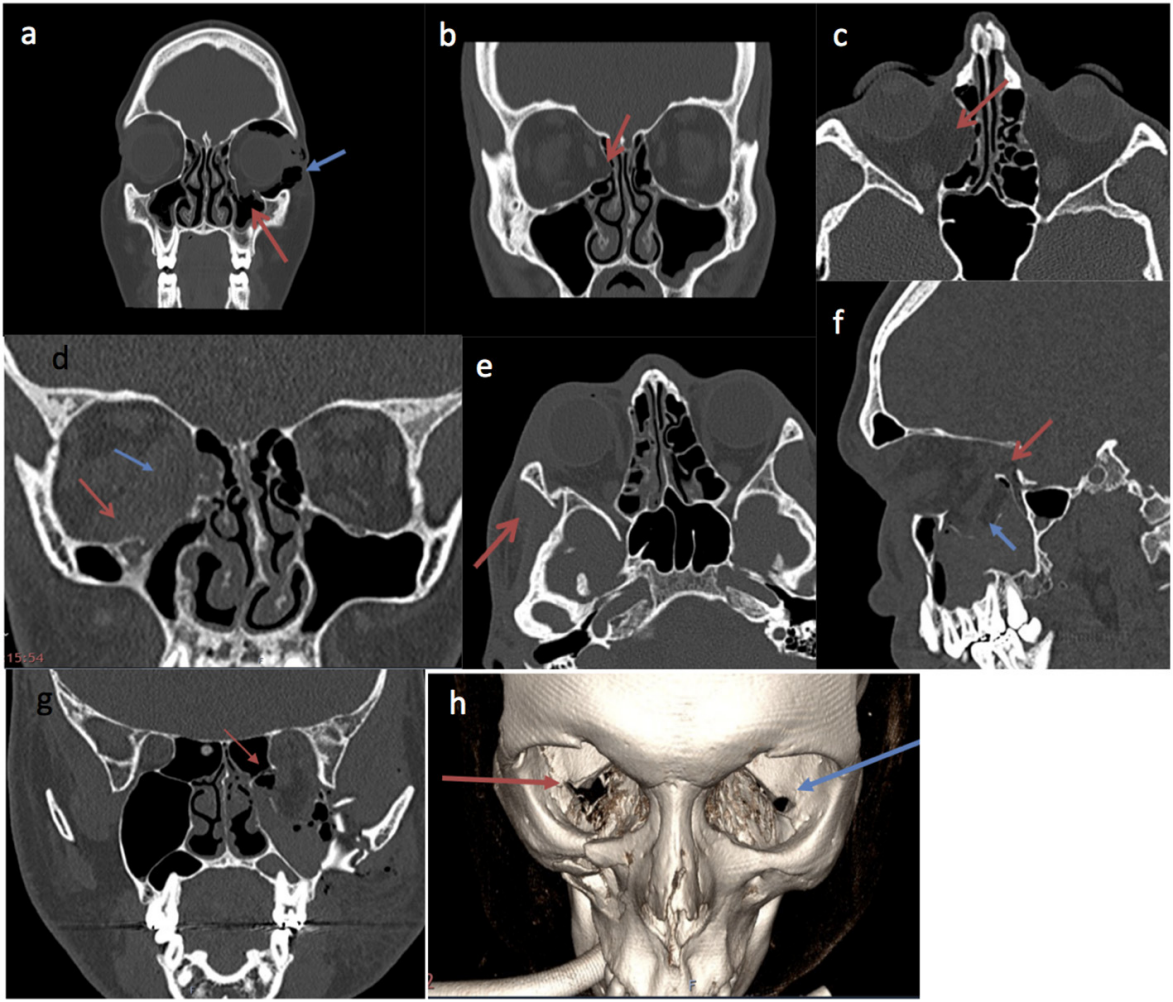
Pre-operative CT imaging examples of fracture parameters used in analysis. (a) Coronal slice of facial bones CT showing an isolated left orbital floor fracture (red arrow) associated with orbital emphysema (blue arrow). (b) Coronal slice of a facial bone CT demonstrating isolated right medial orbital wall fracture (red arrow). (c) Axial slice of a right isolated medial wall fracture on CT orbit (red arrow). (d) Coronal slice of facial bones CT showing right medial wall and right floor fracture. The red arrow points to a right orbital floor fracture. The blue arrow demonstrates right medial wall fracture. (e) Right lateral wall fracture (red arrow) on CT orbits. (f) Sagittal slice of a facial bones CT image demonstrating orbital floor fracture of >50% (blue arrow). The red arrow shows insufficient intact posterior ledge enough to support a plate, making surgical repair more challenging. (g) Coronal slice of facial bones CT showing left orbital floor and medial wall fracture. The red arrow demonstrates the involvement of the posterior medial bulge. (h) 3D image reconstruction of facial bones CT, the red arrow shows the involved inferior orbital fissure of the right orbital fracture. The blue arrow shows the intact left inferior orbital fissure.
